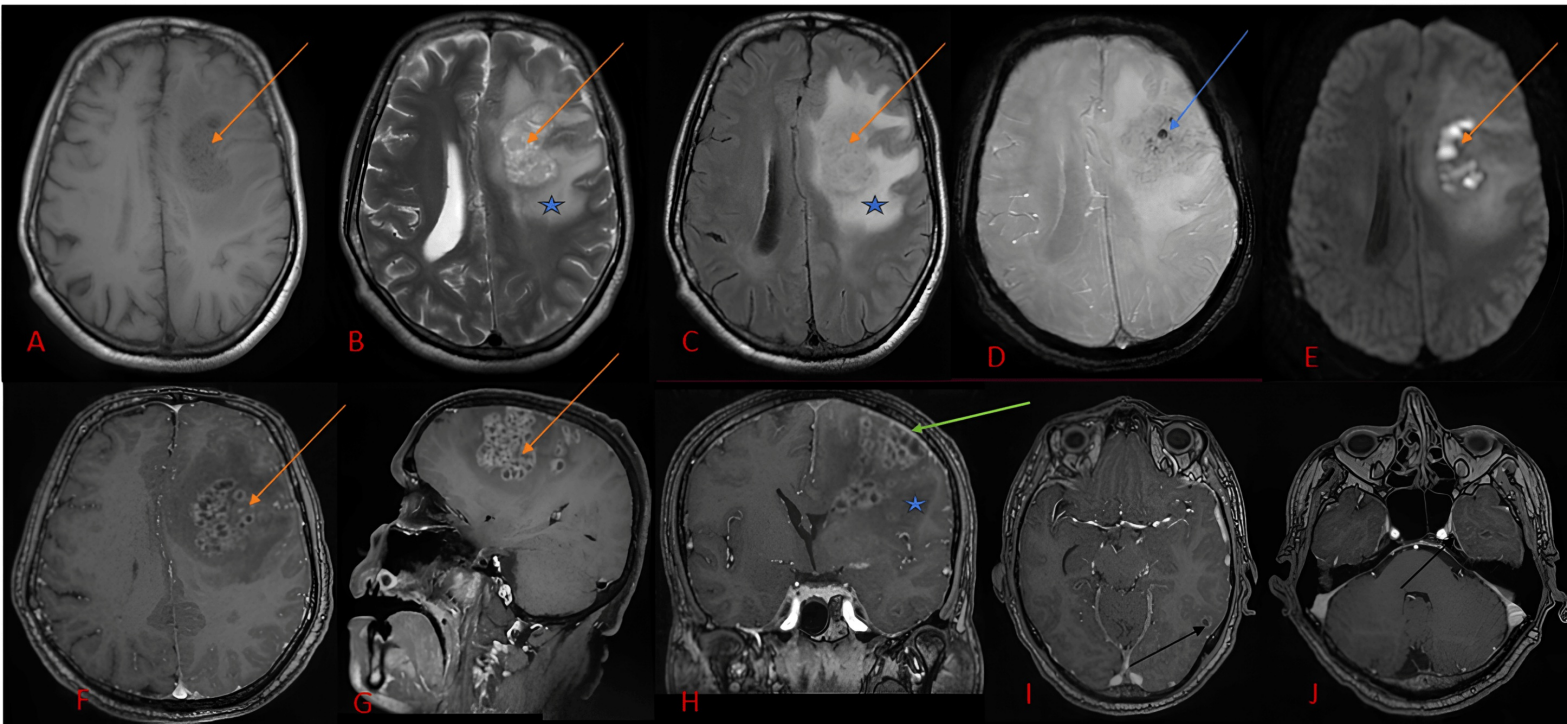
MRI images showing a multi-loculated left frontal lesion (orange arrow) with log contents in T1 hypo signal (A), intermediate signal in T2 and FLAIR (B, C), with diffusion restriction (D), and gadolinium enhancement of the log walls (F, G), with hemorrhagic remodeling in SWI (E) signal void (blue arrow), with individualization of other similar lesions at a distance (black arrow in I, J), peri-lesional edema (star B, C, and H) and a nearby meningeal reaction (green arrow in H) FLAIR: fluid-attenuated inversion recovery; SWI: susceptibility weighted imaging
